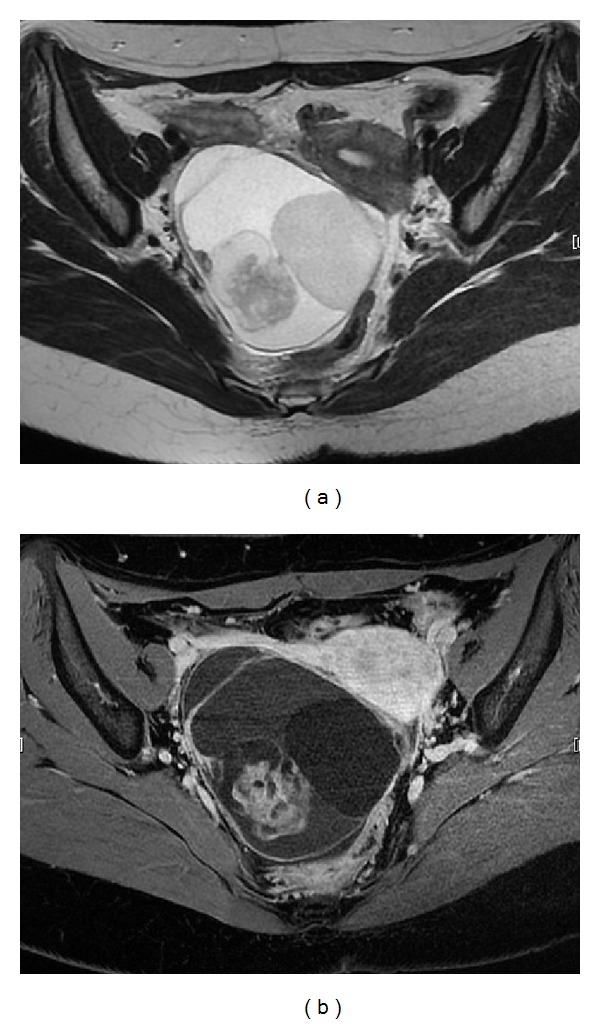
Right ovarian tumor with a solid part in the cystic cavity before the fourth surgery. The tumor size was greater than that before the third surgery. (a) Axial T2-weighted image shows high-signal intensity mass. (b) Gadolinium-enhanced T1-weighted image shows focal enhancement of the tumor.
